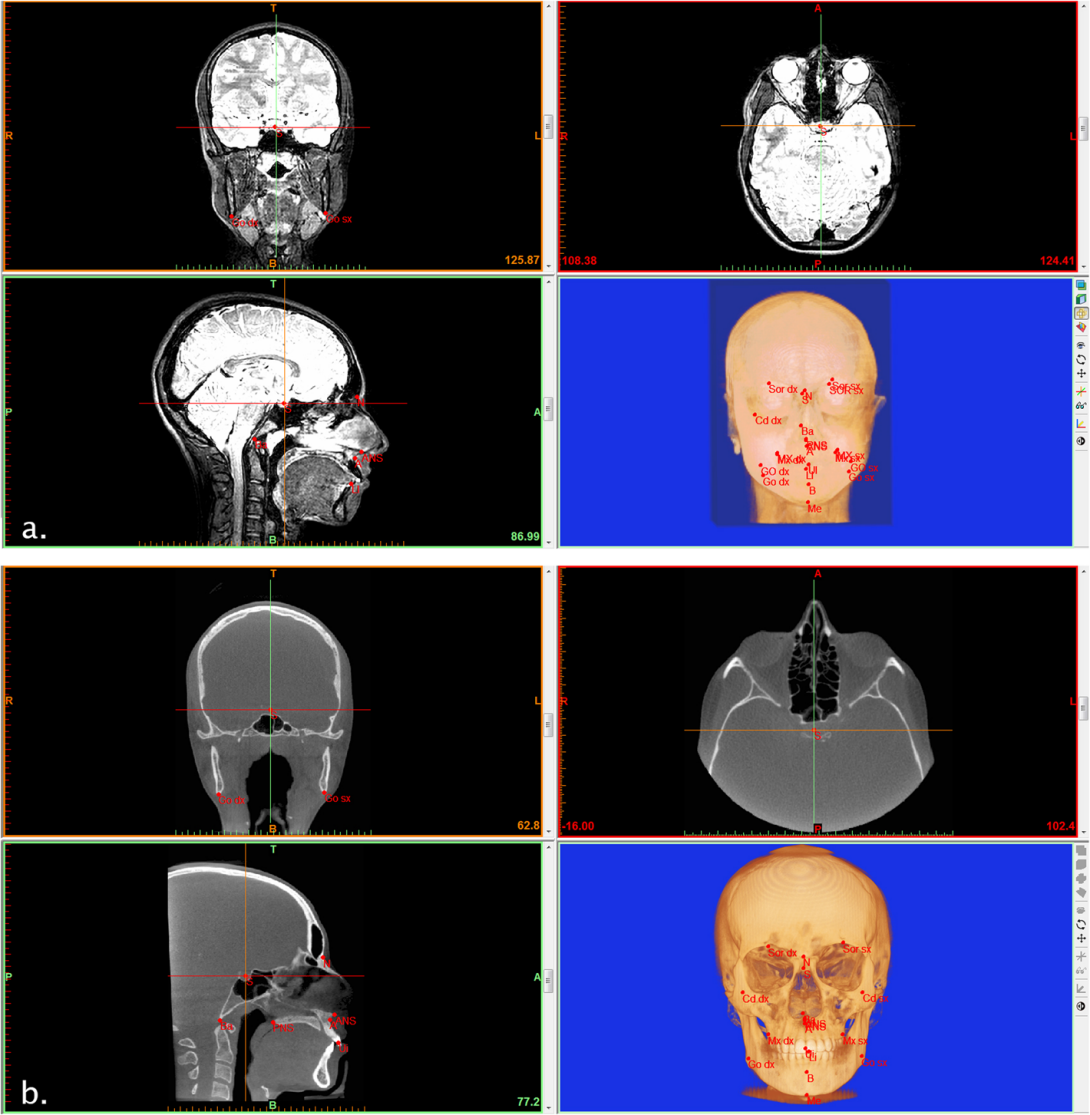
Multiplanar reconstruction and 3D reconstruction views after landmark identification. a Snapshot of software interface, showing 3T-MRI cephalometric landmarks on axial, coronal, and sagittal sections and on 3D volumetric rendering. b Snapshot of the software interface, showing CBCT cephalometric landmarks on axial, coronal, and sagittal sections and on 3D volumetric rendering
